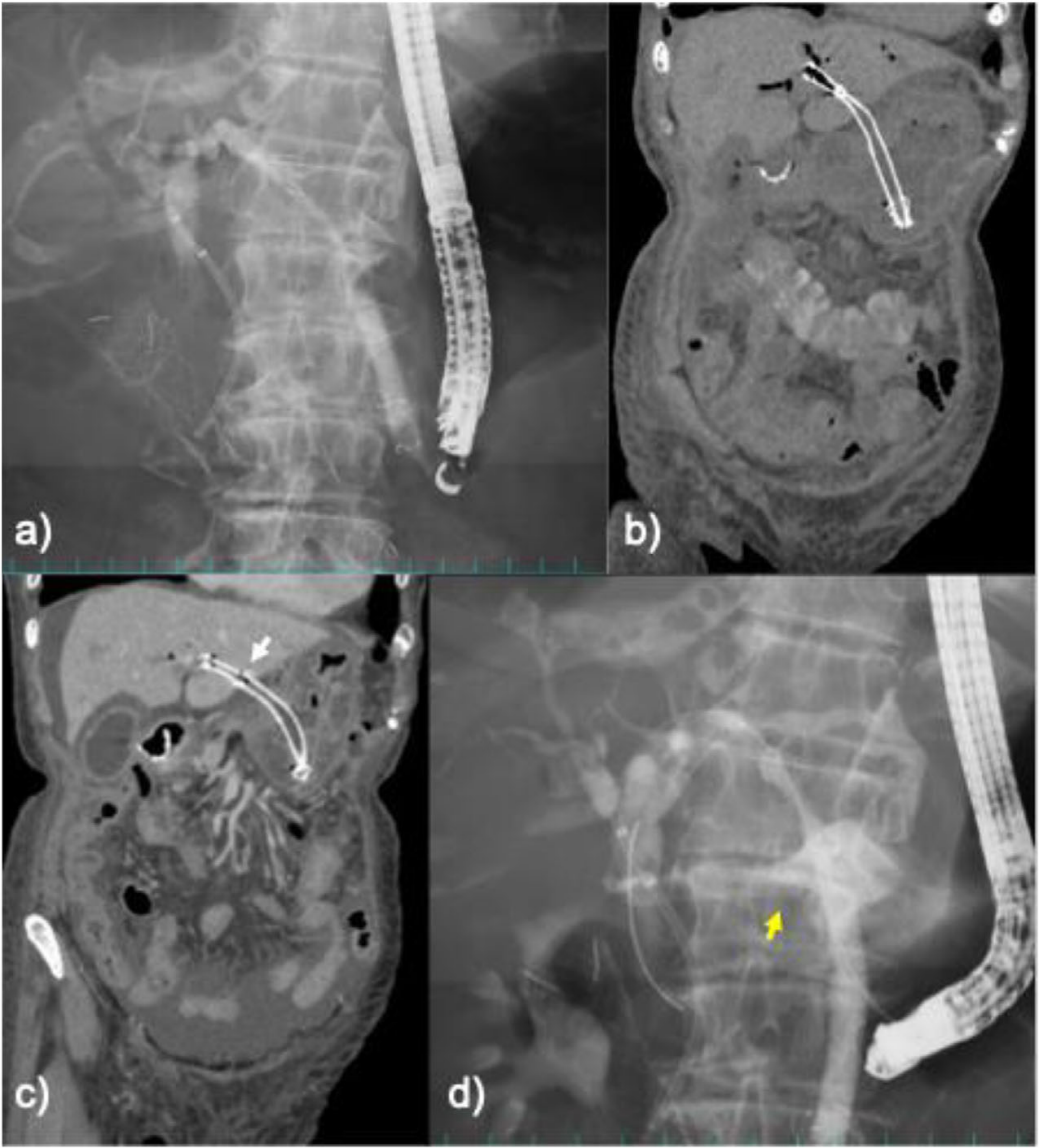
Imaging findings after endoscopic ultrasound‐guided hepaticogastrostomy (EUS‐HGS) and subsequent stent migration. (a) Endoscopic ultrasound‐guided hepaticogastrostomy (EUS‐HGS) with placement of a partially covered self‐expandable metal stent (SEMS) from the B3 duct to the stomach. (b) Postoperative computed tomography confirmed the correct positioning of the partially covered SEMS (PCSEMS) for EUS‐HGS. (c) Three days later, computed tomography demonstrated a slight, distal dislocation of the SEMS on the hepatic side, with the marker between the uncovered and covered portions falling outside the liver margin (white arrow). (d) Cholangiography during the reintervention demonstrated contrast leakage from the uncovered segment of the SEMS into the peritoneal cavity (yellow arrow).
